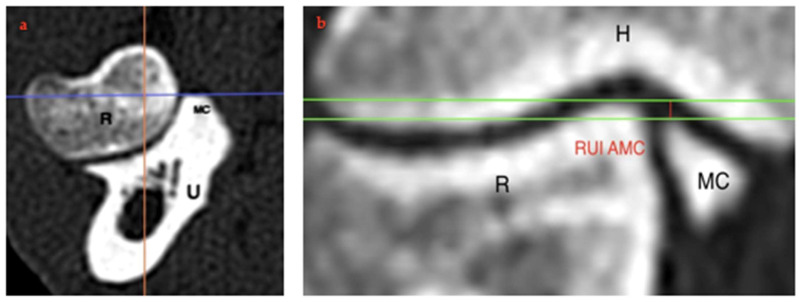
(a) Transverse plane of the radio-ulnar joint for localization of the AMC (blue line) using the centre line (orange line) to be assessed. (b) Reformatted CT images in the dorsal plane. Exemplary representation of the change in RUI AMC (red line) in the neutral position (0°). H: humerus, R: radius, U: ulna, MC: medial coronoid.
